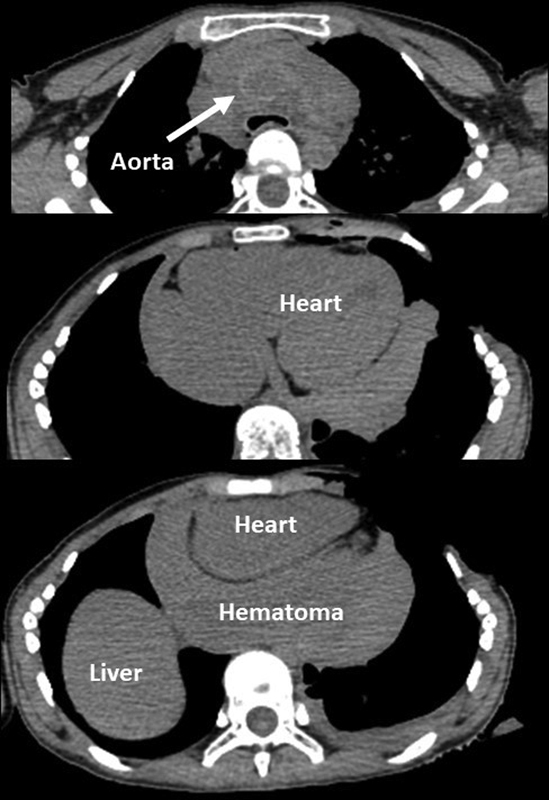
Autopsy imaging. Computed tomography indicated residual pericardiac hematoma.
